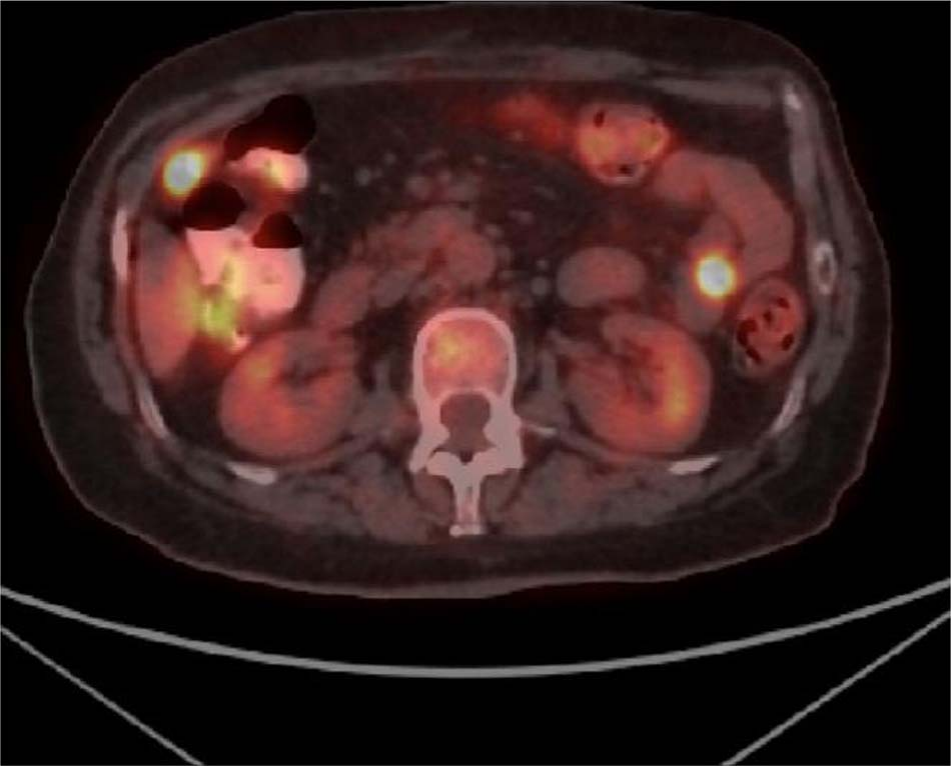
PET CT showing metabolic activity in abdominal and pelvic lymph nodes.
